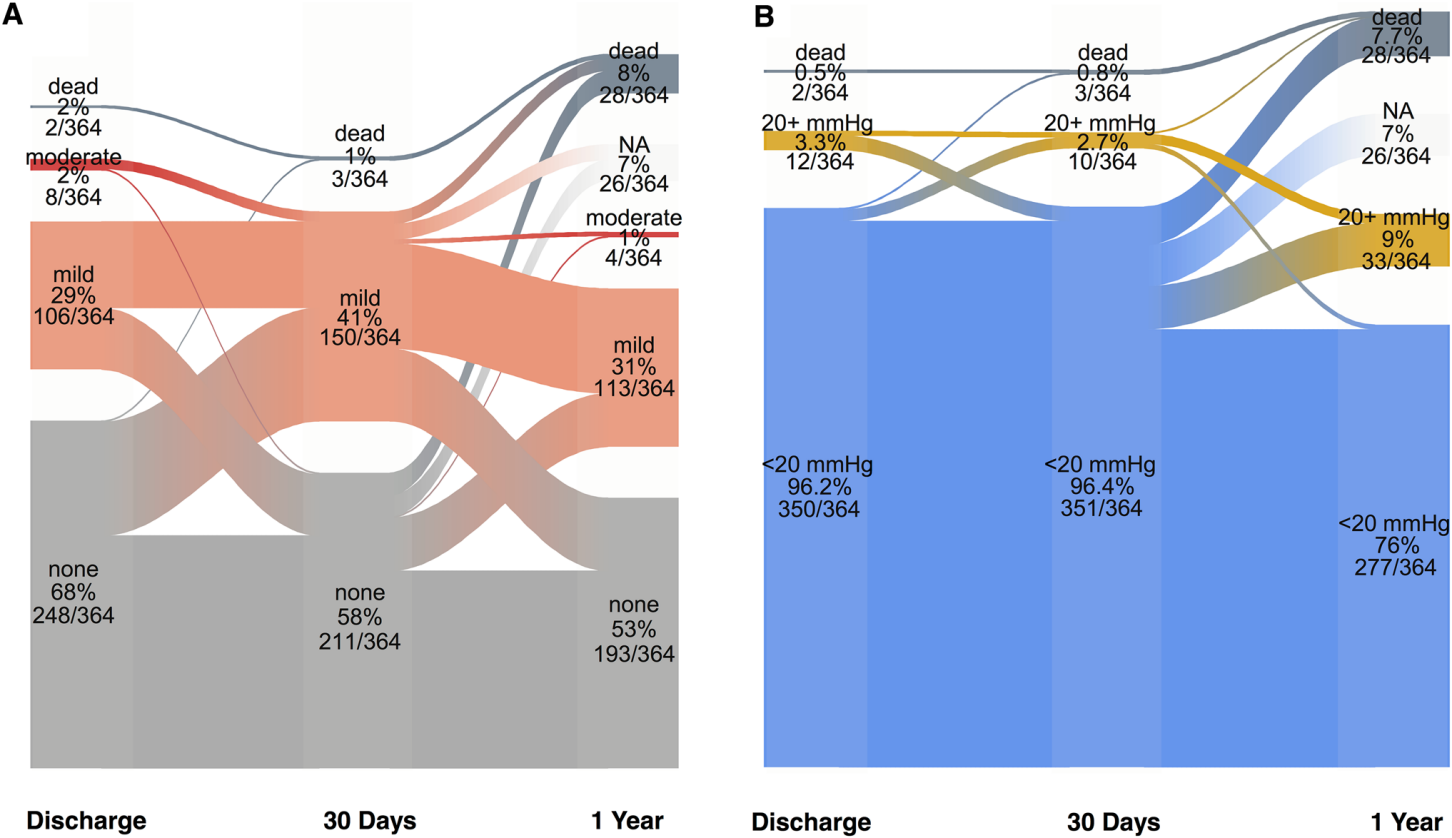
Echocardiographic valve performance after discharge for PVL (a) and transvalvular gradients (b). Change in PVL (a) and elevated gradients during the first year after TAVI. Note that only patients with complete echocardiography at discharge and 30 days or dead (*n* = 364) are displayed
